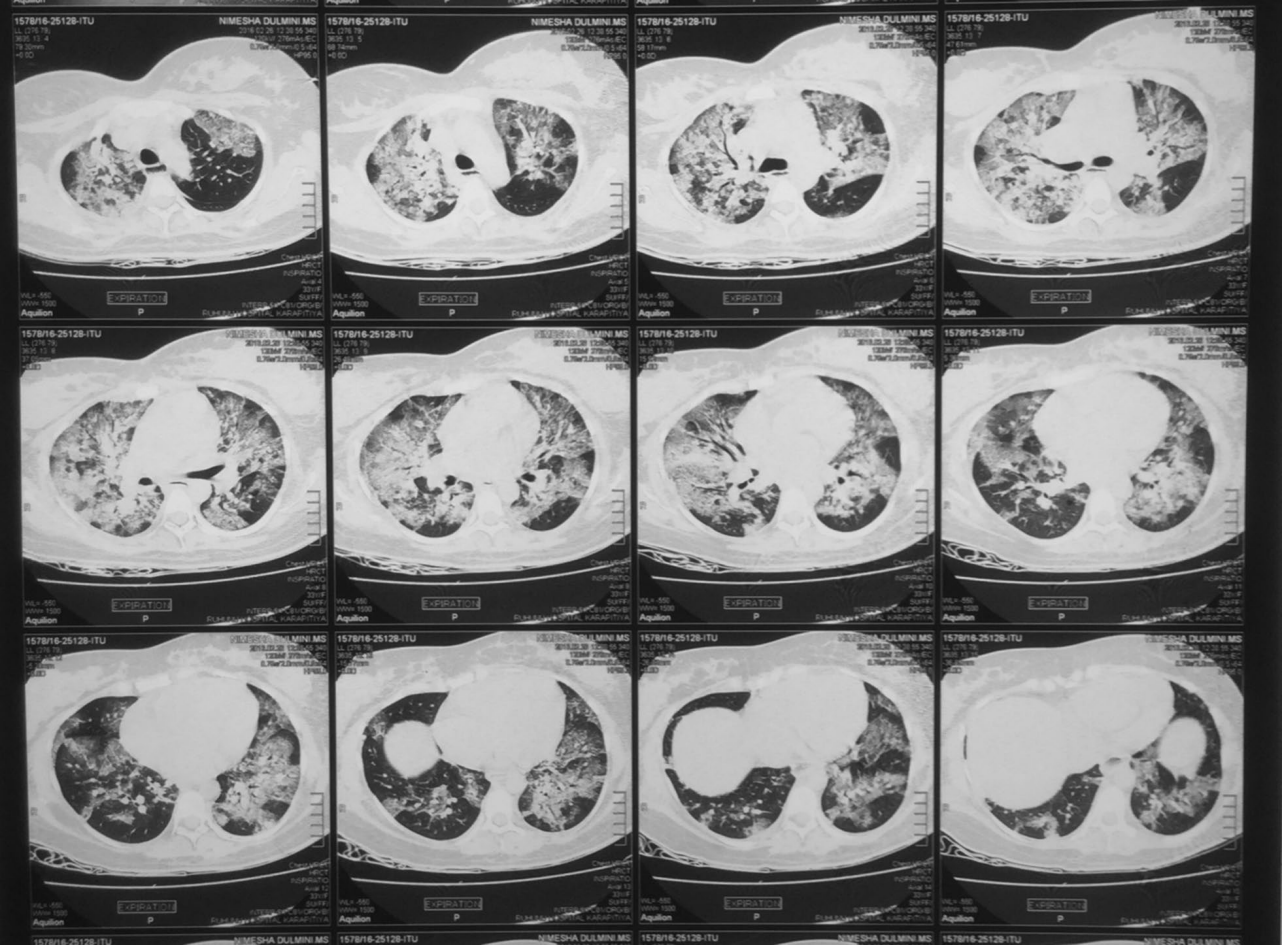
High resolution computed tomography of chest. Shows ground glass opacities throughout bilateral lung fields with multi lobar consolidation
